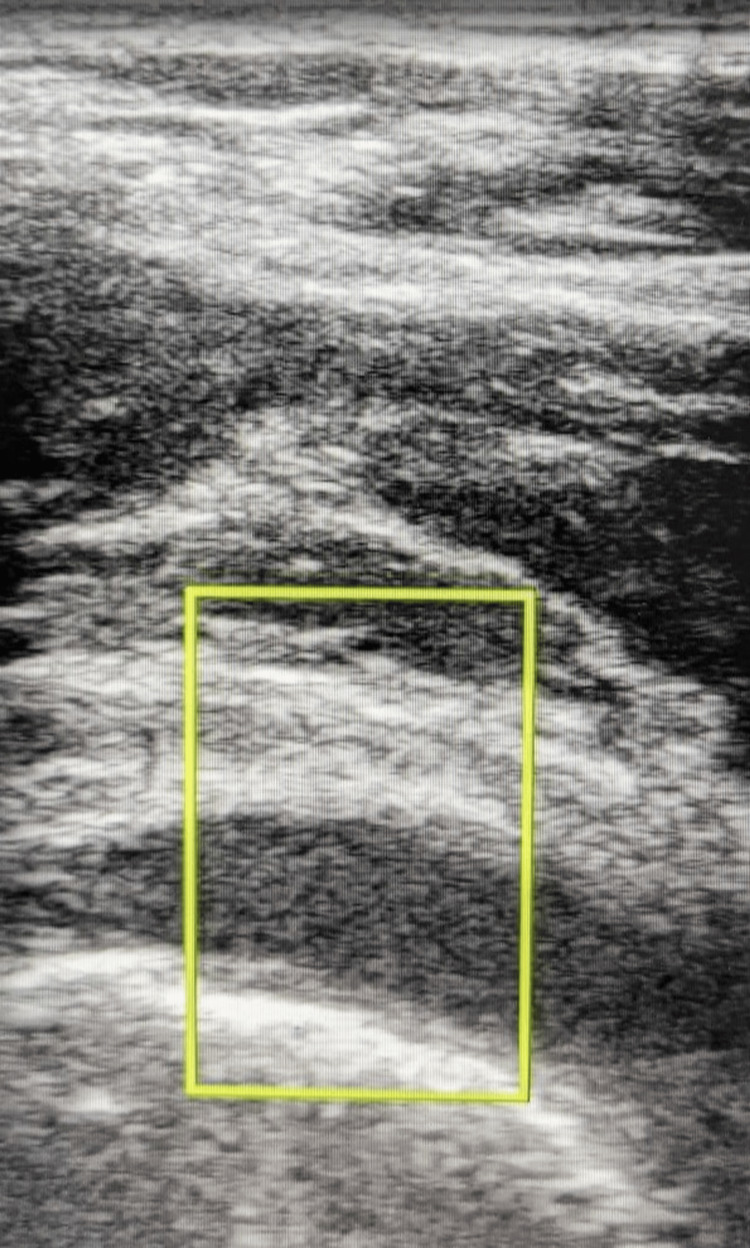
Hip Effusion Seen on Ultrasound The effusion is the hypoechoic (darker grey and black area) seen just above the hyperechoic (bright white) curvature of the femoral head toward the bottom third of the image. Color Doppler, which would be found inside the yellow box, was used over the effusion area. Since there was no color flow seen, it was less likely to be a hypoechoic area due to vascular etiology. There was also no evidence of color flow immediately surrounding the hypoechoic area that might suggest a rim-enhancing abscess.
